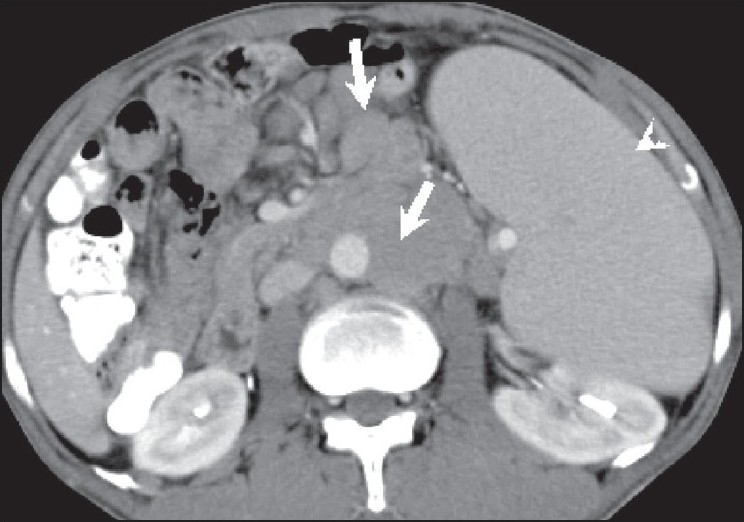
Lymphoma. A 45-year-old male with non-Hodgkin lymphoma has para-aortic and mesenteric lymphadenopathy (arrows) along with splenomegaly (arrowhead), on a contrast-enhanced, axial CT scan of the abdomen
